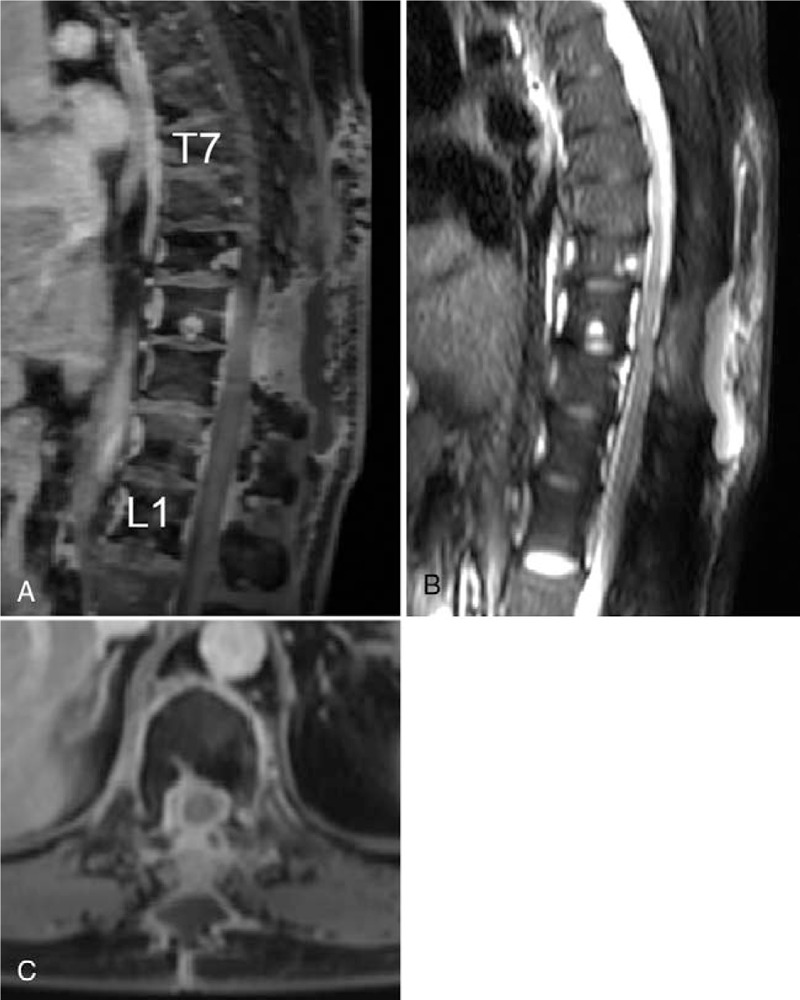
Preoperative T1 gadolinium-enhanced (A and B), T2-weighted (C) sagittal MR images, and axial images at the level of T10 showing a decompression of the cord and remaining mass lesion.
